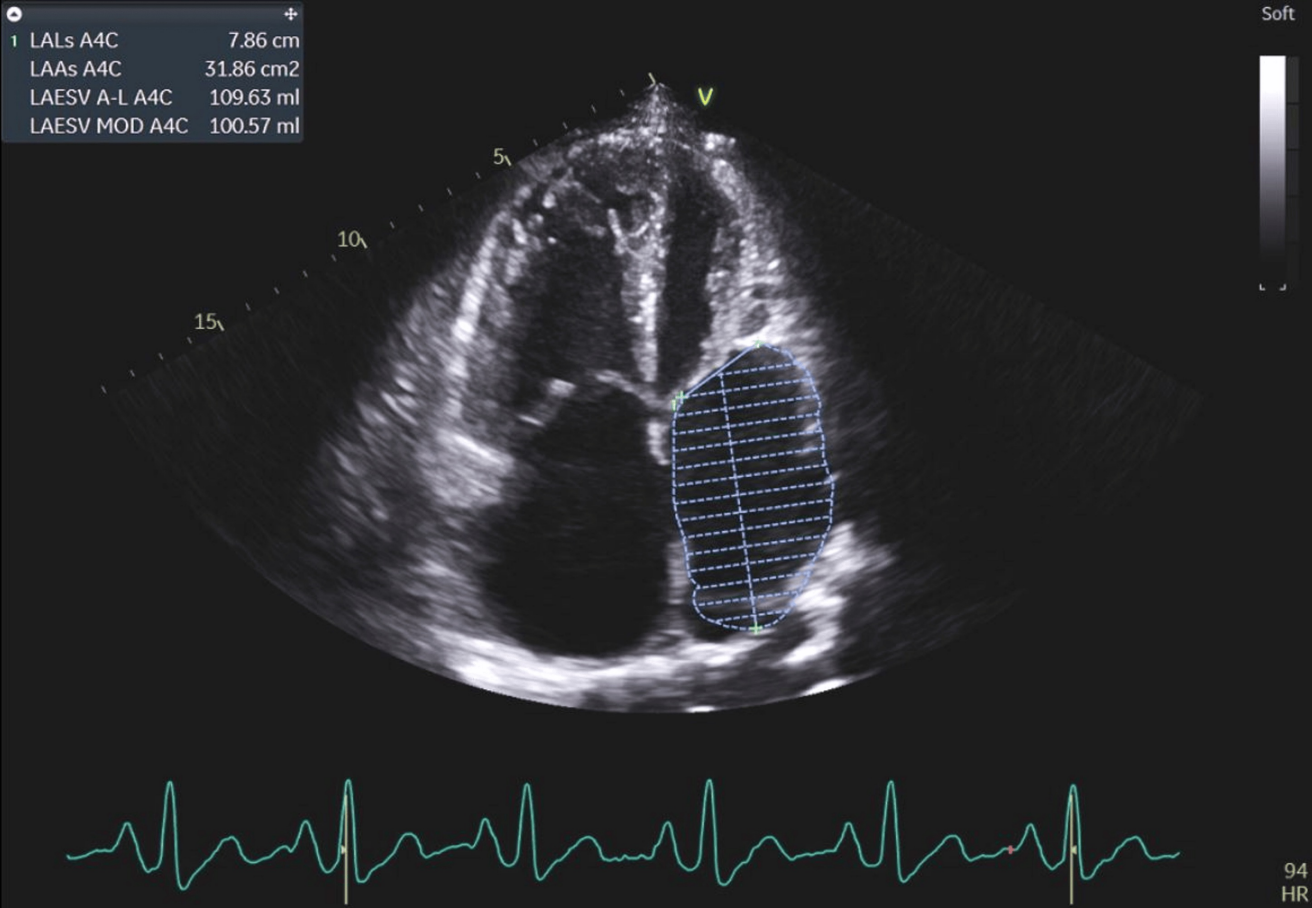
The patient's left atrial enlargement secondary to coexisting minor stenosis and mitral valve regurgitation, both of which cause chronic left atrial volume-pressure overload. LALs A4C: left atrial length, systolic, apical 4 chamber; LAAs A4C: left atrium area, systolic, apical 4 chamber; LAESV A-L A4C: left atrial end-systolic volume, area-length, apical 4 chamber; LAESV MOD A4C: left atrial end-systolic volume, method of discs, apical 4 chamber
